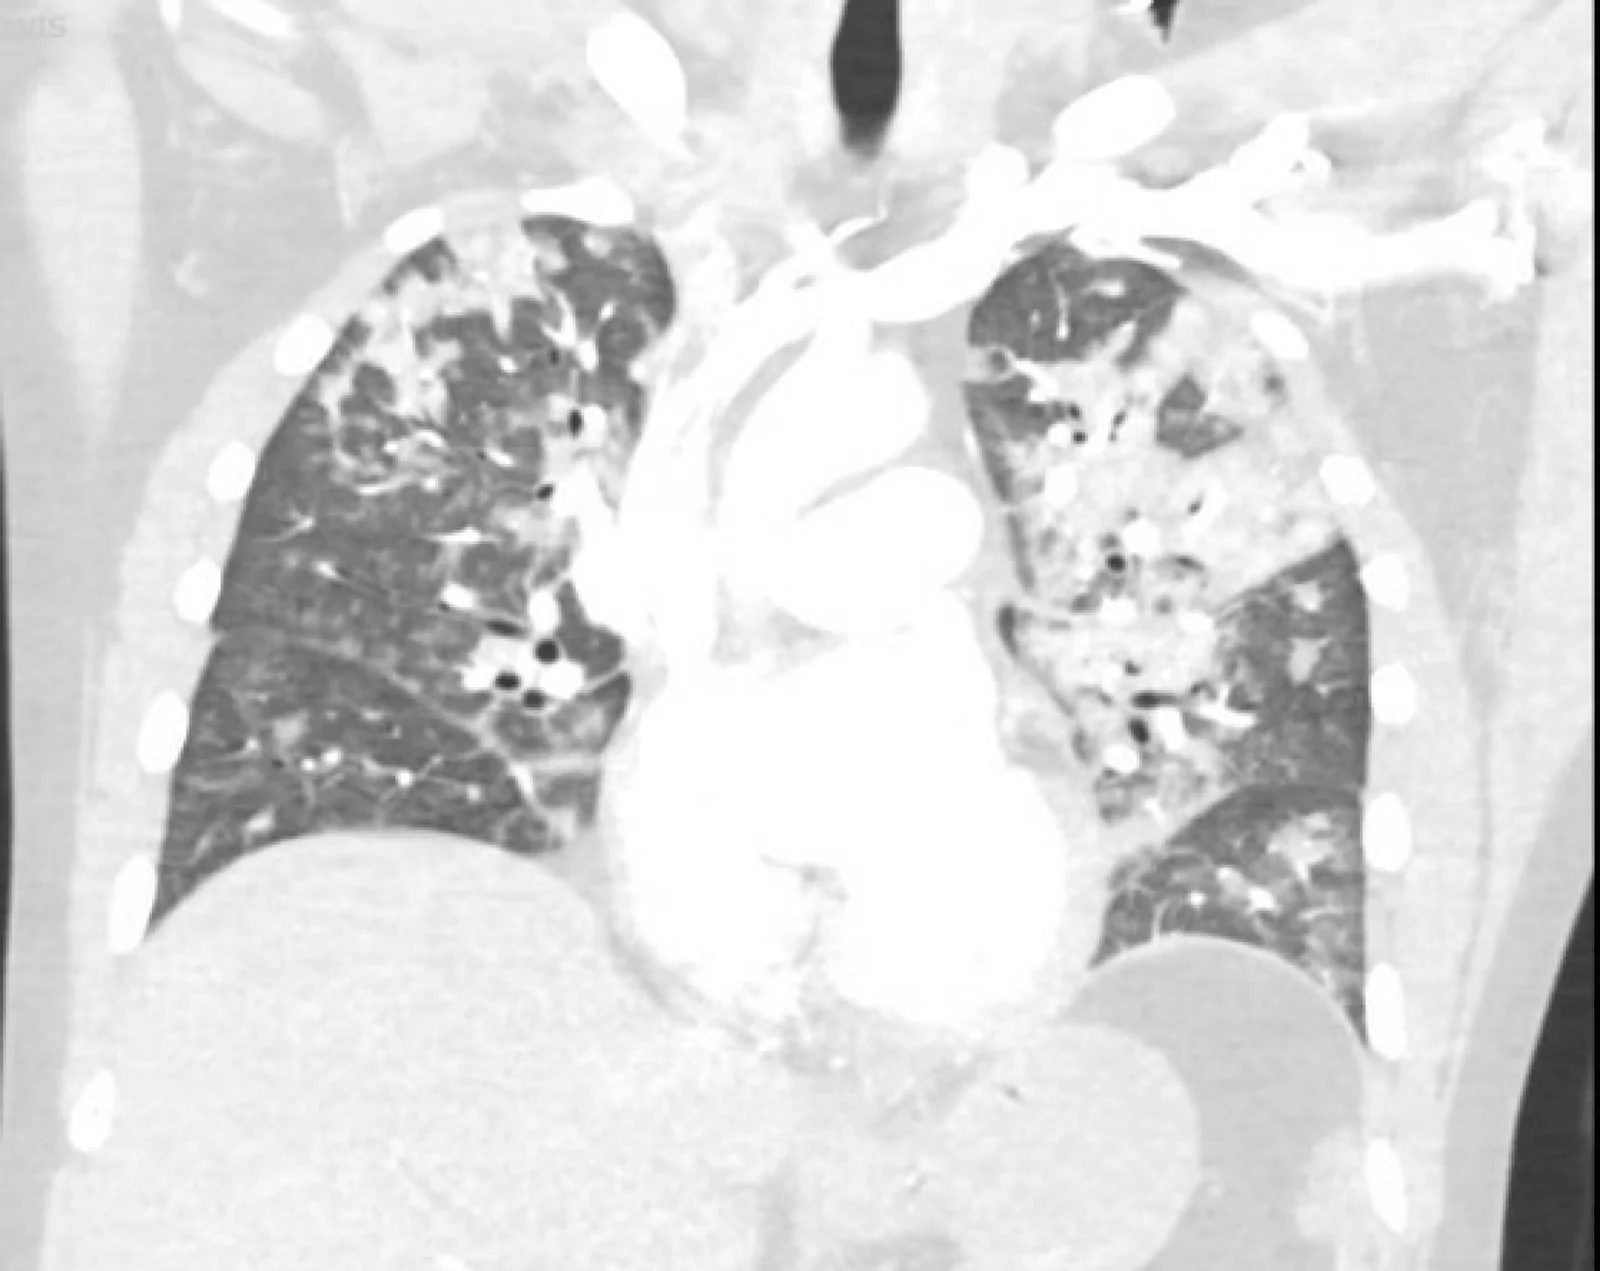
Computed tomography angiography of the chest. Coronal computed tomography angiography of the chest demonstrating bilateral multifocal ground-glass and consolidative pulmonary opacities involving both lungs, with greater involvement of the upper lobes. No evidence of pulmonary embolism was identified. Findings were consistent with pulmonary edema and acute lung injury in the setting of severe amphetamine-induced cardiomyopathy.
